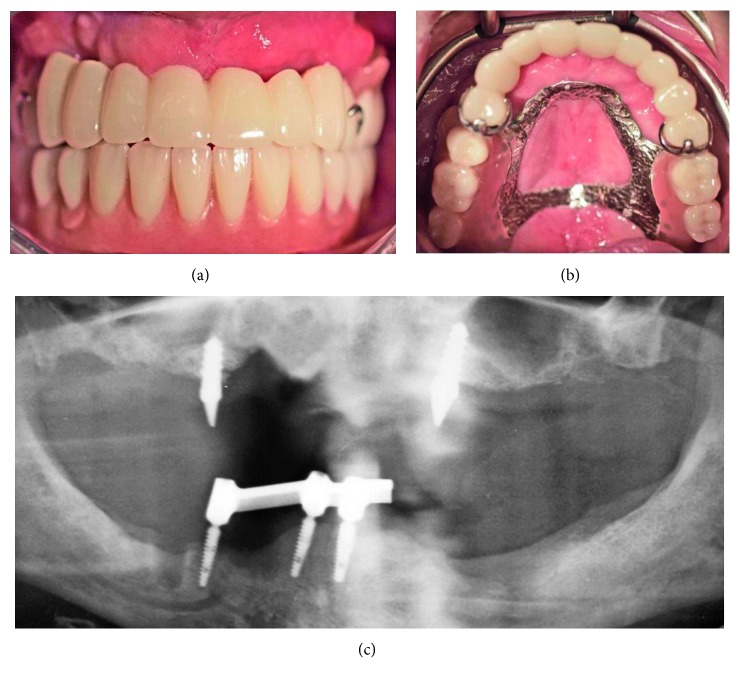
(a, b) Maxillary temporary rehabilitation with FPD retained on implants #4 and #10 and removable denture for the molar areas. (c) OPG 4 weeks after surgery with the mandibular overdenture (not modified radiograph taken during current treatment).
